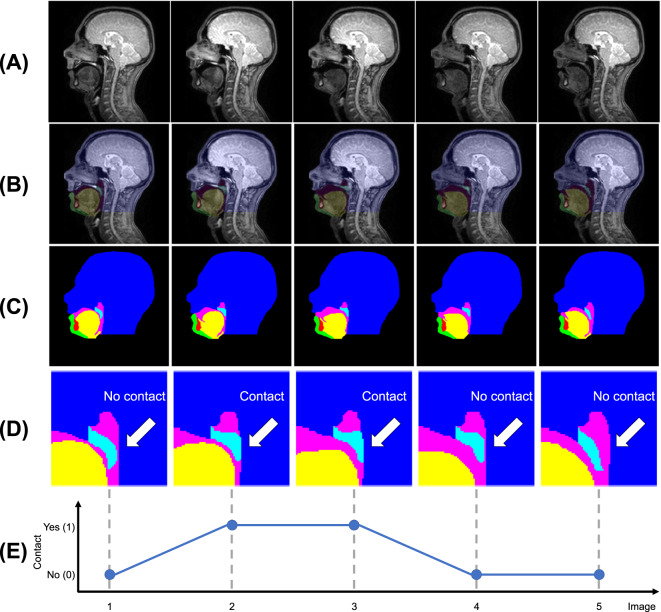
Five consecutive images from one of the magnetic resonance image series (A), corresponding ground-truth (GT) segmentations overlaid on the images (B), GT segmentations only (C), GT segmentations cropped around the soft palate with labels indicating if there is contact between the soft palate and posterior pharyngeal wall (D), and a line chart indicating if there is contact (Yes) or not (No) between the soft palate and posterior pharyngeal wall in each image in the series (E). The GT segmentations are of the head (dark blue), soft palate (light blue), jaw (green), tongue (yellow), vocal tract (pink) and tooth space (red) classes.
